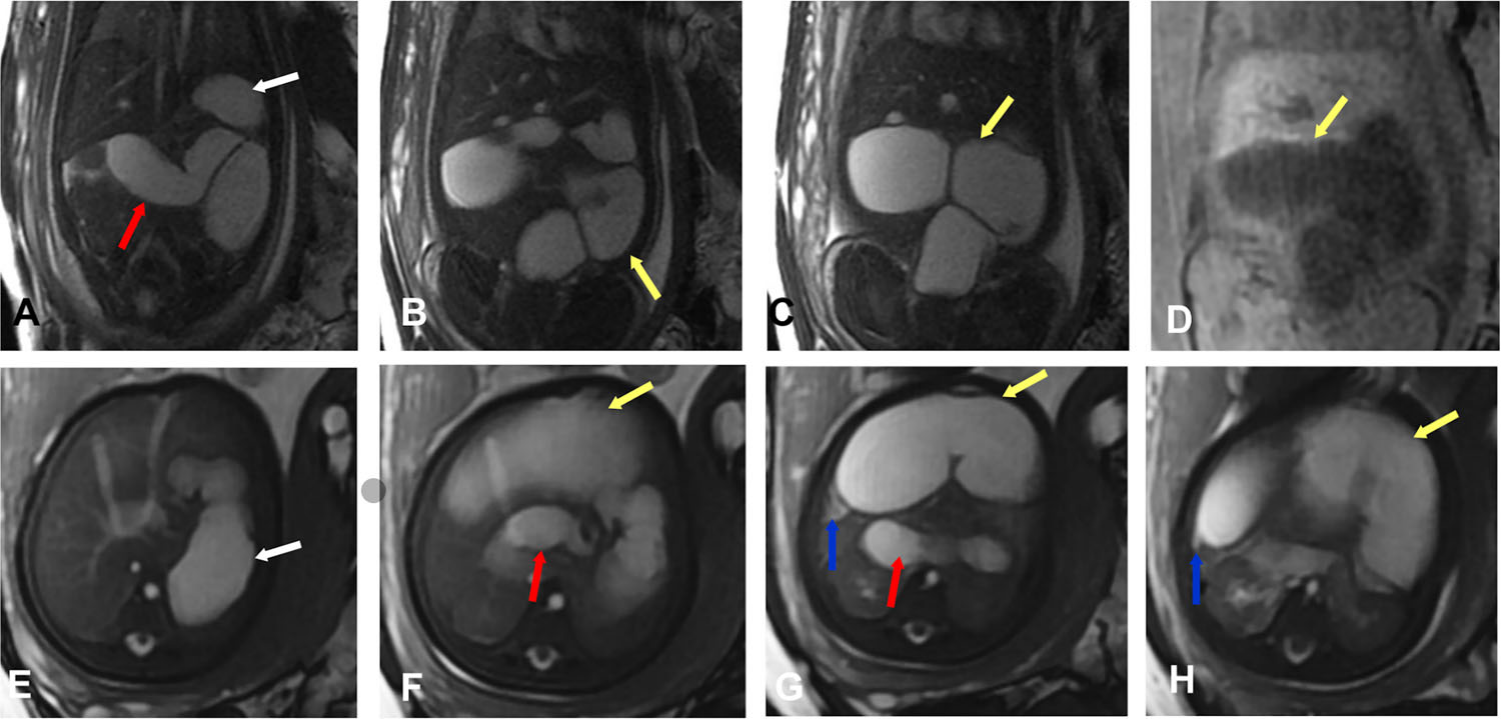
Jejunal atresia. Ultrasound: intestinal obstruction. Gestational age 32 weeks and 6 days. A, B Coronal FIESTA. C Coronal 3D LAVA. D Coronal FIESTA. E–G Axial FIESTA. H Axial cine FIESTA. Dilation of the stomach (white arrows), duodenum (red arrows), and especially the proximal jejunum (yellow arrows) up to the hepatic flexure, demonstrating hypointense signal on T1 and hyperintense on T2. A sudden change in caliber of the jejunal loop is identified at the hepatic flexure due to jejunal atresia (blue arrows)
